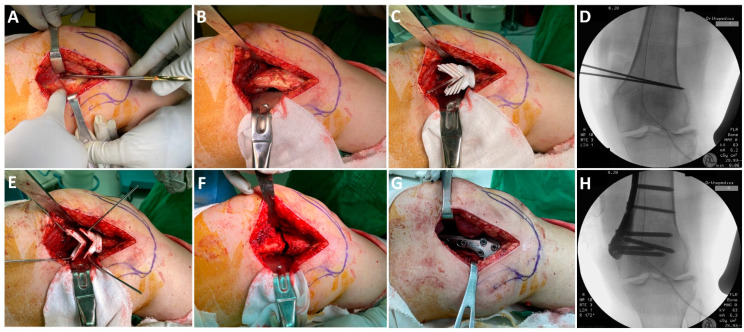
The surgical approach and the intraoperative radiographs. (A) A standard medial approach to the distal femur was used, utilizing the plane between the vastus medialis and adductors (distal is to the right and proximal is to the left). (B) The periosteum was elevated from the target femoral surface. The posterior femoral structure was retracted with a Bennett retractor in protection of the neurovascular bundle. (C) The customized cutting jig was placed onto the medial cortex, identifying the ideal spot with an anatomical fit of the guide. Two orientation K-wires were then inserted through the K-wire holes at the apexes of the chevron cut. (D) Positioning of the orientation K-wires was confirmed with a C-arm. (E) Four K-wires were inserted, fixing the guide onto the bone. Osteotomy was performed with a graduated oscillating saw. (F) The cutting guide and the wedge were removed after the osteotomy. (G) The osteotomy was then closed with gentle manual bending and fixed with the associated locking plate system. (H) The final position of the plate was confirmed with a C-arm. An alignment rod was not used.
